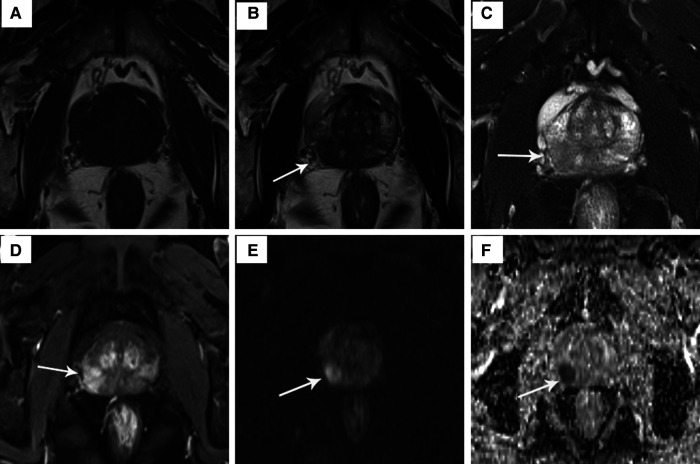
The signal characteristics of a 64-year-old PCa patient on T1W image, T2W image, T2W enhancement image, DWI and ADC map. (A) T1W image: no obvious discovery; (B) T2W image: mild hypointensity in the prostate's intermediate right posterolateral zone (arrow); (C) hypointensity on T2W fat suppression sequence; (D) T2W enhancement image: hyperintensity confirmed presence of lesion; (E) hyperintensity on DWI (*b* = 800 s/mm^2^); (F) hypointensity on ADC map.
